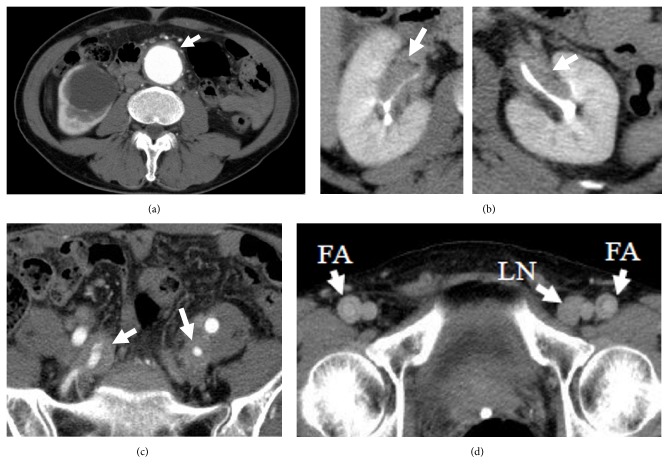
CT scan when the patient first came to our hospital. (a) The abdominal aorta was enlarged, and the adventitia was thickened. (b) Soft tissues around the ureters were thickened. (c) The tissue around the bilateral internal iliac arteries was thickened. (d) The adventitia of the bilateral femoral arteries was thickened and lymph nodes were swollen. CT, computed tomography; FA, femoral artery; LN, lymph node.
